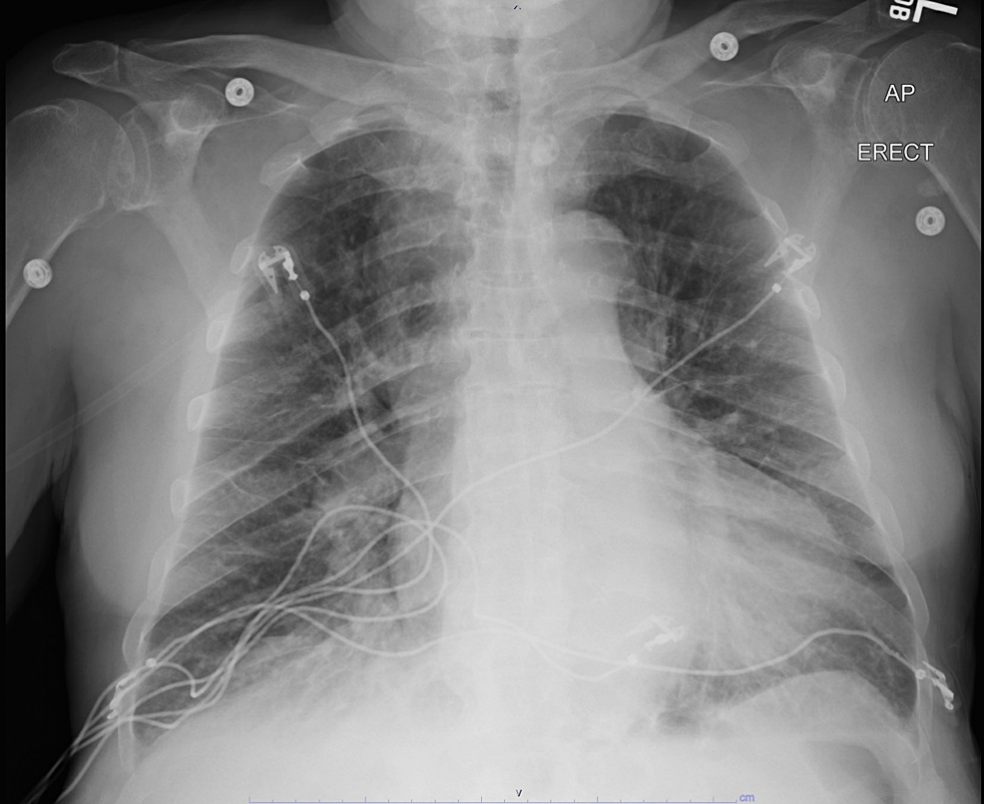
Chest X-ray after the development of pulmonary edema. AP: Anteroposterior
